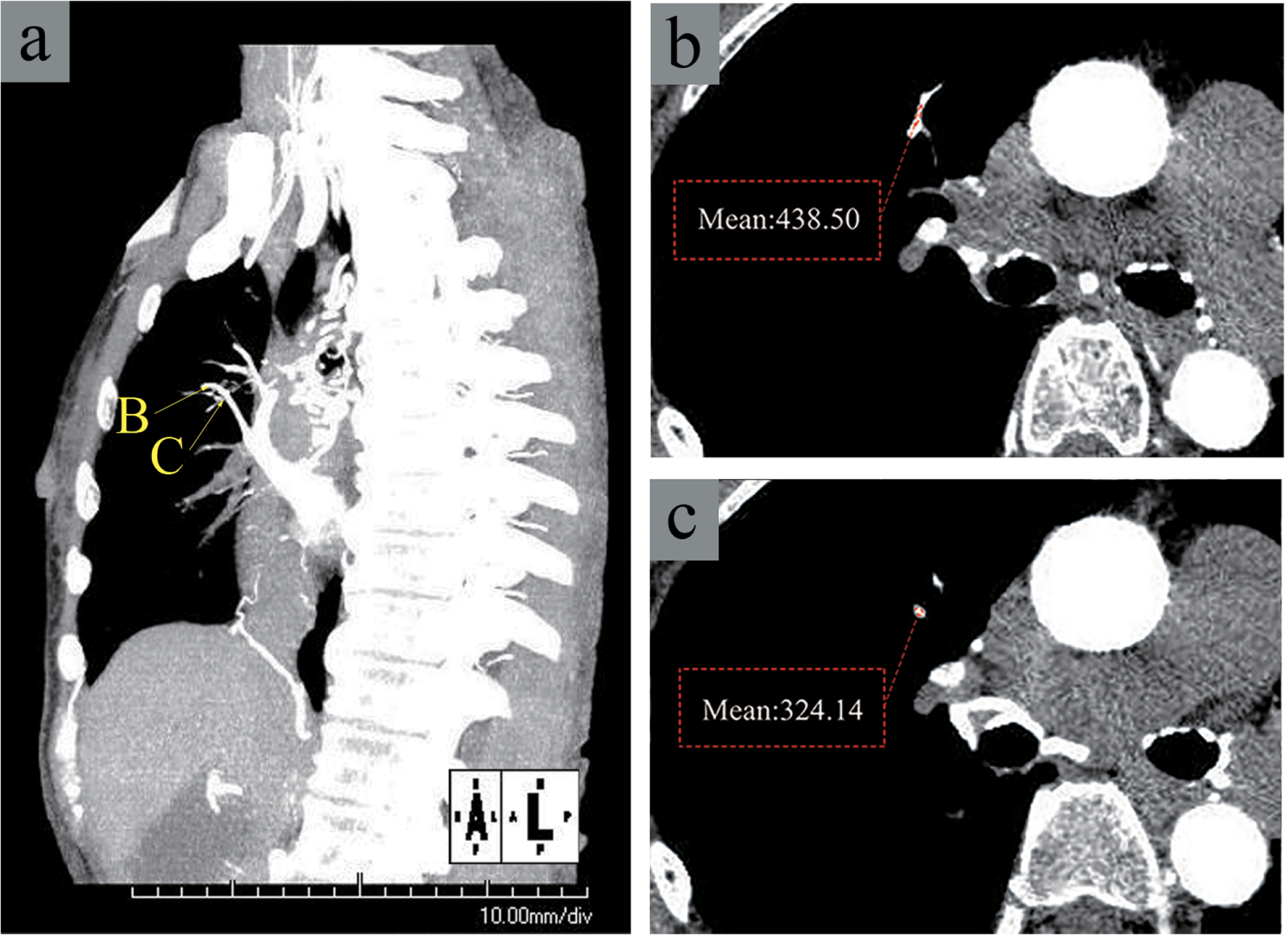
Enhanced pulmonary veins shown on intra-aortic computer tomographic angiography in a representative patient (72-year-old woman with chronic thromboembolic pulmonary hypertension). a Left anterior oblique view (60 degrees) MIP image with 20 mm slice thickness showing enhancement in the pulmonary veins of the right S3; arrows B and C indicate slice levels of the corresponding original transverse images shown in b and c, respectively. Transverse images at the levels corresponding to arrows B and C, respectively, in the image shown in a. The numbers indicate mean CT values (HU) of ROIs placed on the segmental pulmonary vein (V3). The mean of the mean CT values was regarded as the HU-PV for the right V3
